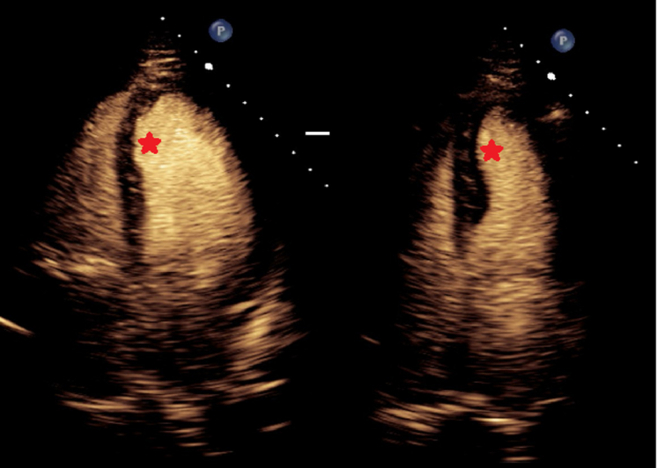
Transthoracic Echocardiogram With Contrast to Assess for Wall Motion Abnormality (Left) End-systole and (Right) end-diastole images with a hypokinetic segment of the apical septal wall and apex (red stars).
